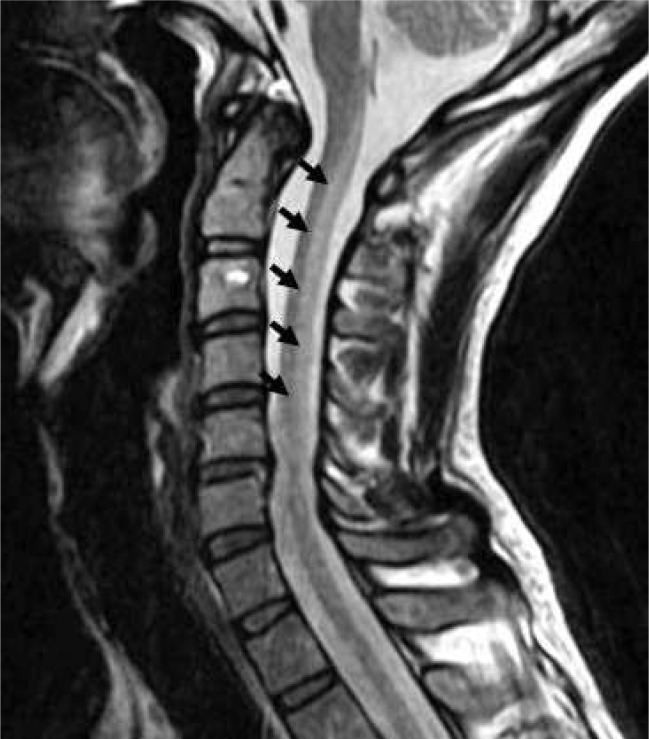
Sagittal T2 weighted MRI showing long (C2- C7) hypersignal lesion in posterior part of spinal cord (black arrows)
